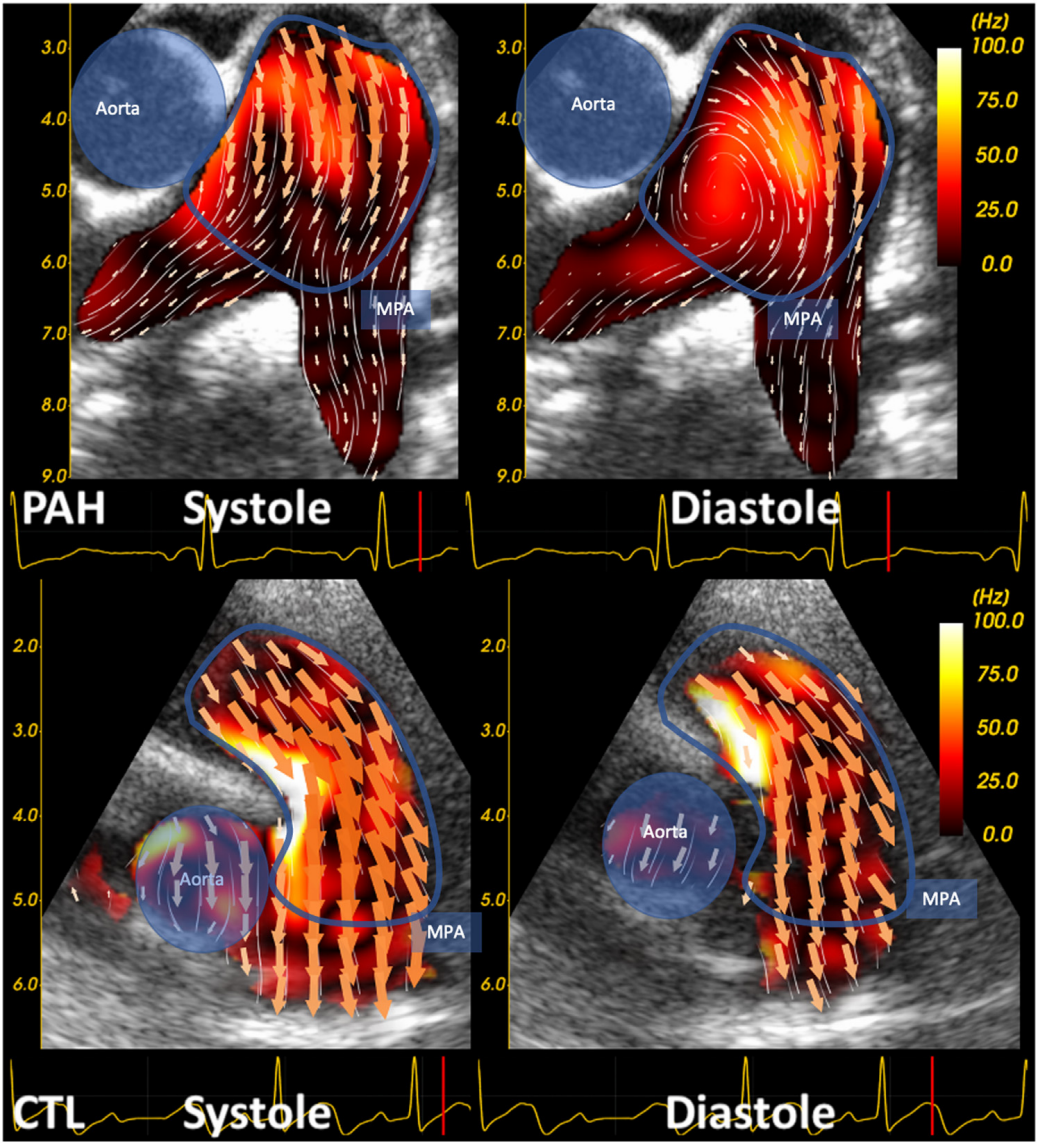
Typical main pulmonary artery (MPA) flow patterns as shown by high-frame-rate ultrasound imaging and blood speckle tracking. The vorticity map is seen in the background. CTL, control; PAH, pulmonary arterial hypertension.
